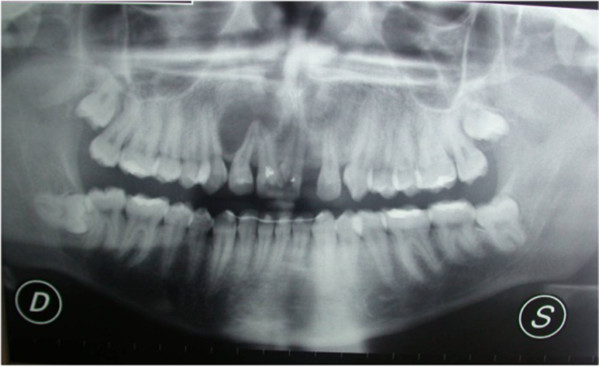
Panoramic radiographic.
